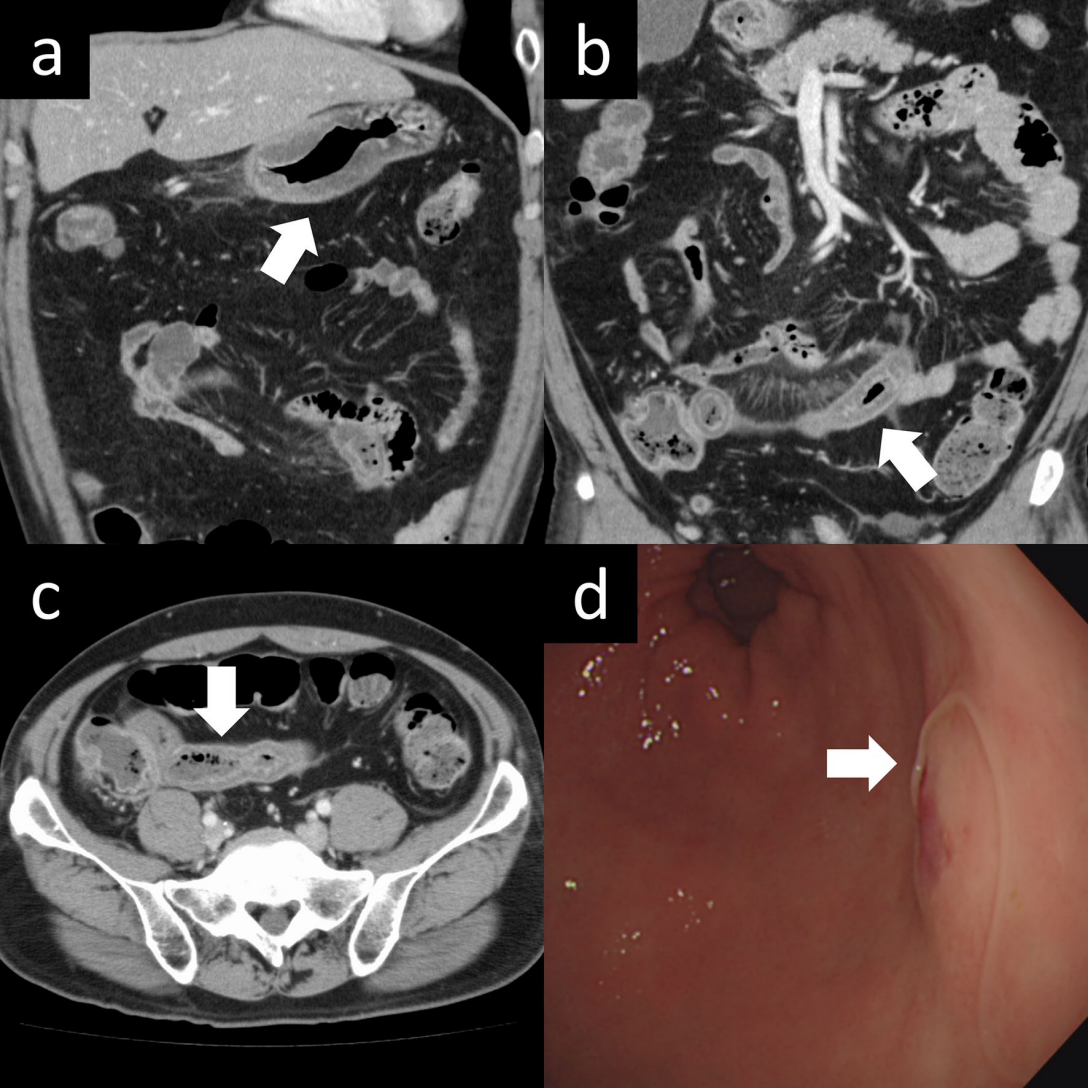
A 55-year-old man presented to the emergency department with a complaint of abdominal pain since the day before. Contrast-enhanced CT images in the coronal plane demonstrate edematous wall thickening of the stomach and increased density of the surrounding fat tissue (Fig. 3a, arrow). Similar edematous wall thickening and increased fat density are seen in the small intestine and colon at the ileocecal region on coronal and axial contrast-enhanced CT images (Fig. 3b, C, arrows). An *Anisakis* larva was identified and removed during upper gastrointestinal endoscopy (Fig. 3d, arrow)
